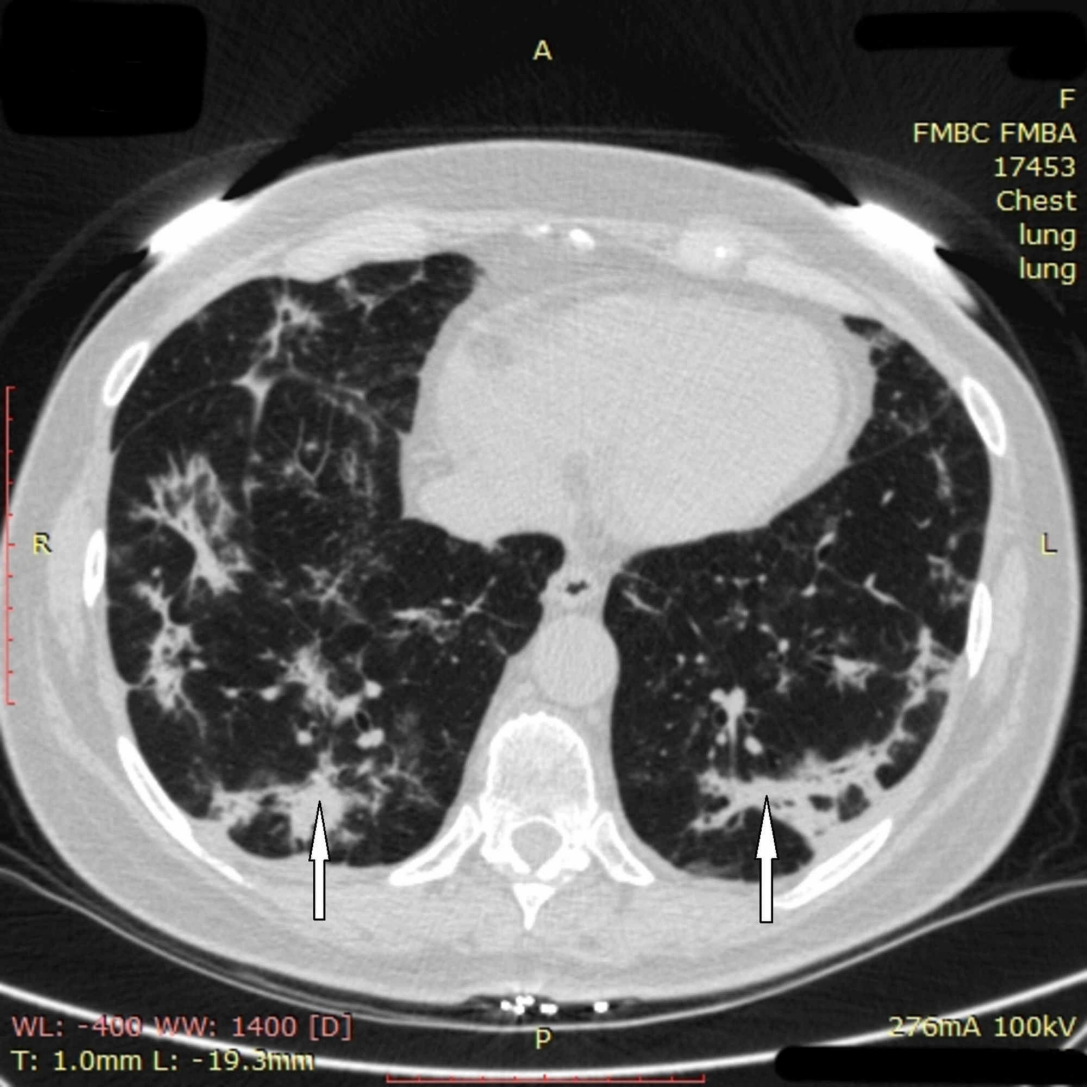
Chest CT imaging of patient 2 on day 13 Chest CT on day 13 showed partial or complete resolution of the opacities in both lungs with the amount of involved pulmonary tissue decreased to less than 50%. A portion of opacities in all segments of the lungs had turned into consolidation (white arrows) CT: computed tomography
